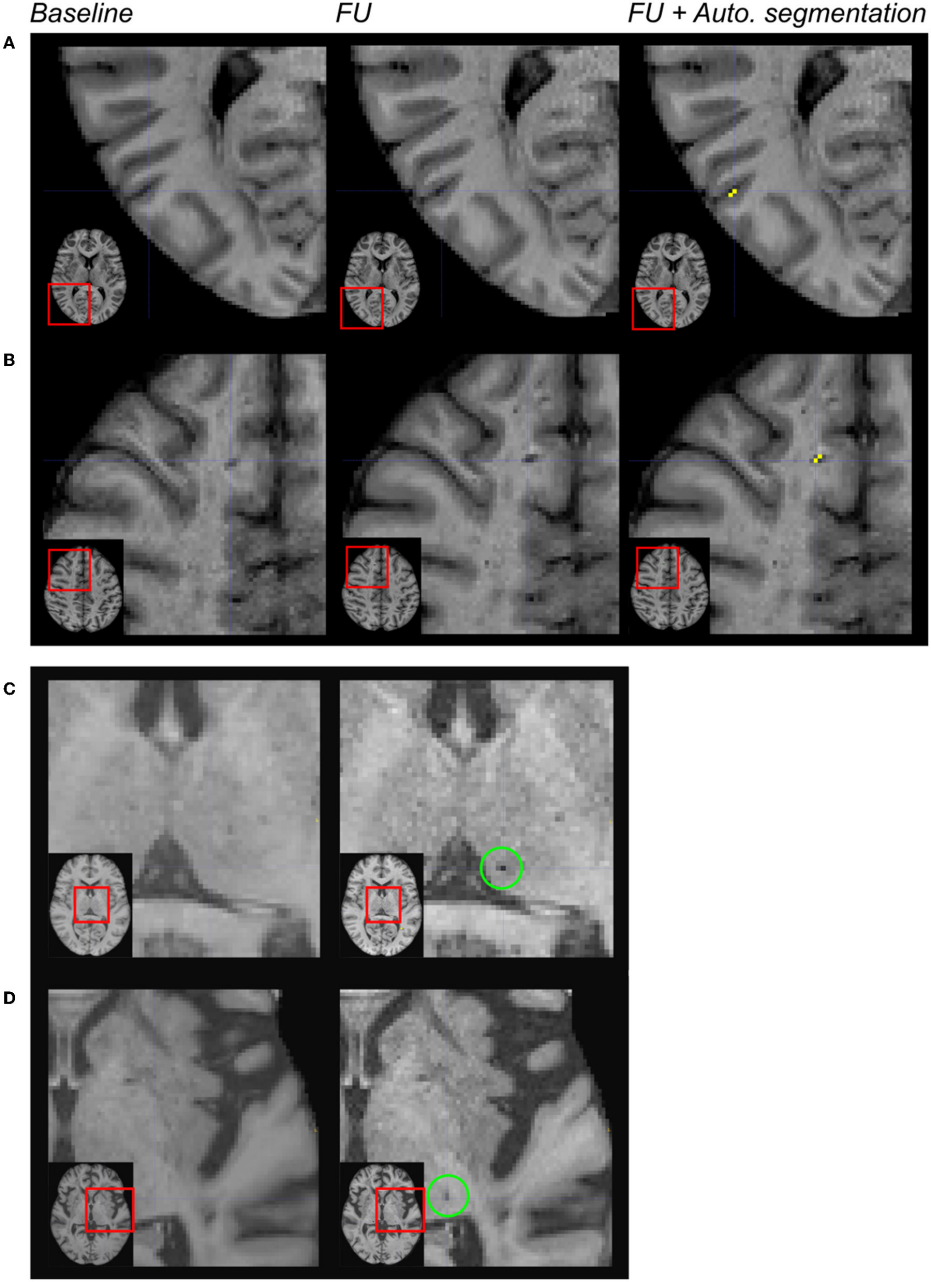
Limitations of the automated segmentation. From left to right: magnification of MR images in different patients after the registration of the baseline and follow-up (FU) data in the MNI space. In the right column, the automated segmentation of lesions was superimposed on the FU MRI. Because of brain atrophy in patients with CADASIL, false positive lacunes were identified by the algorithm after the enlargement of some cortical sulci (A). Because the CSF signal has changed across acquisitions within perivascular spaces, false positive incident lacunes were also detected at such locations (B). The algorithm failed because of the size of the cluster of interest (diameter < 3 mm) (C). The algorithm failed because the region of interest did not belong to the CSF mask of follow-up data (D).
